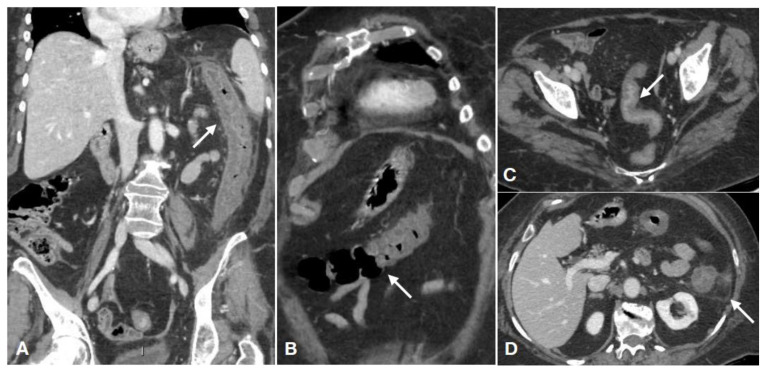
Enhanced CT of a 50-year-old female presenting with abdominal pain and rectorrhagia showing typical CT findings of ischemic colitis. (A,B) Portal-venous phase in coronal view shows continuous thickening of the colonic wall with mucosal hyperemia, submucosal edema and reduced lumen ((A–D), arrows). (B,C) There is involvement of the whole left colon, with a clear distinction between the affected and unaffected segment ((B), arrow), and the coexistence of pericolic fat stranding ((C), arrow). (D) In the axial plane is clearly evident the “little rose” sign ((D), arrow). These findings are consistent with ischemic left colitis with reperfusion. No significant vessel findings were detected.
